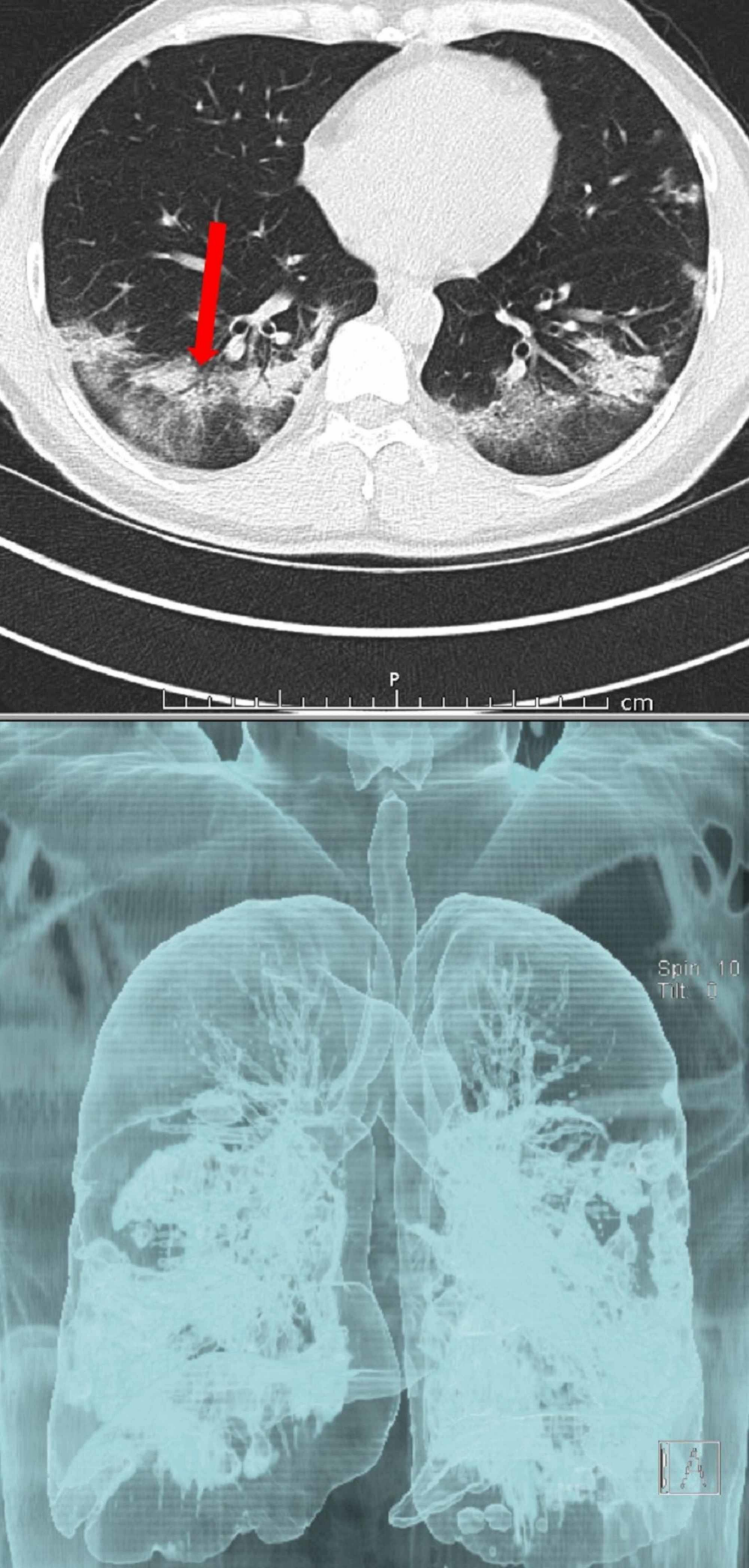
CT examination of the same patients 12 days later Air bronchograms and fibrosis were developed. Bilateral milimetric central aeration areas on 3D image. Sectional routine image better shows lesion details, and 3D images better depict involvement area in the lung. CT, computed tomography
